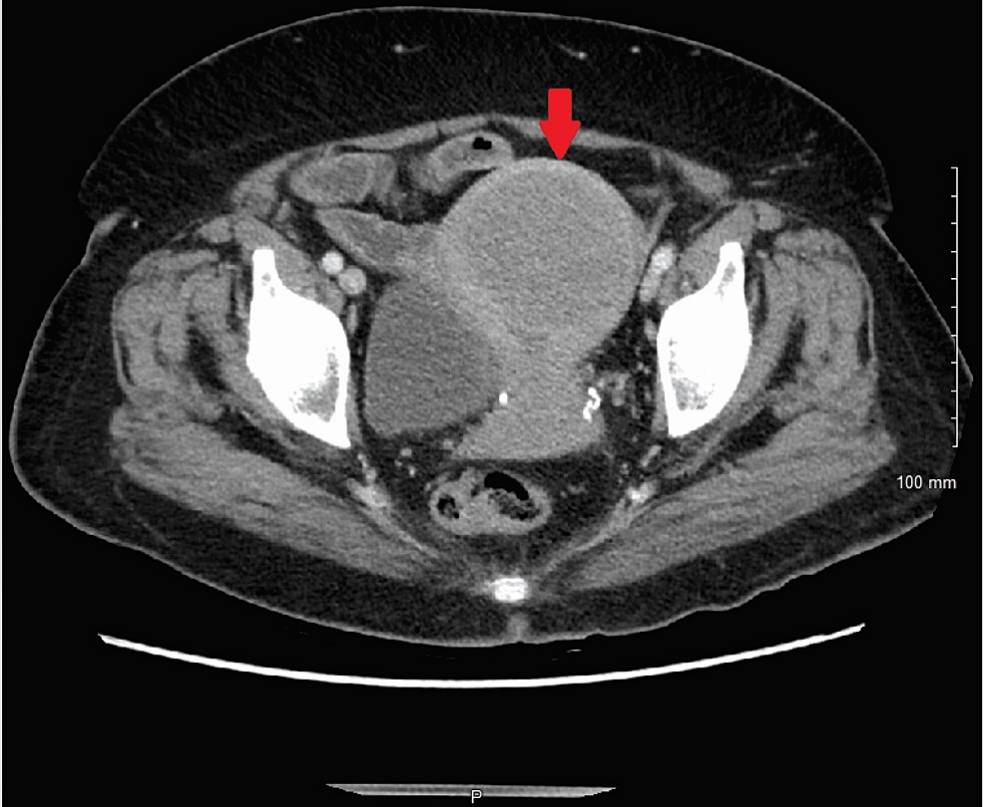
Hypodense nodule approximately 5.6 cm × 6.2 cm in size noted on computed tomography of the abdomen and pelvis, likely a uterine fibroid.
